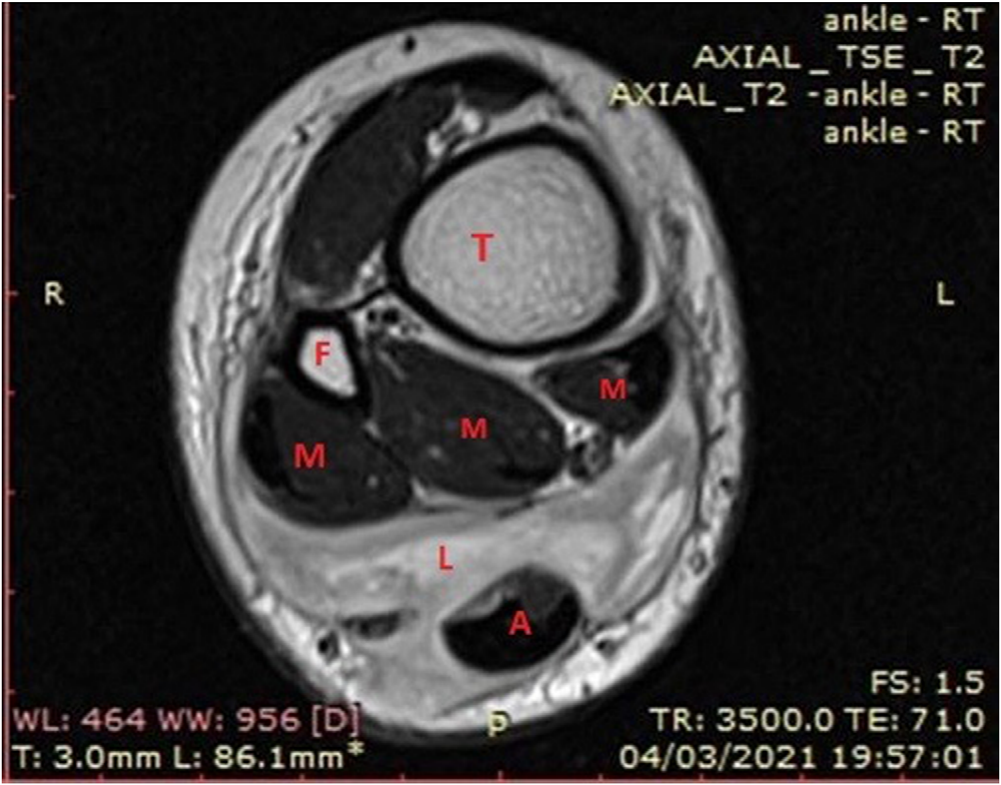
MRI R lower limb: T = tibia, F = fibula, M = muscle, L = lymphoma, A = Achilles tendon
